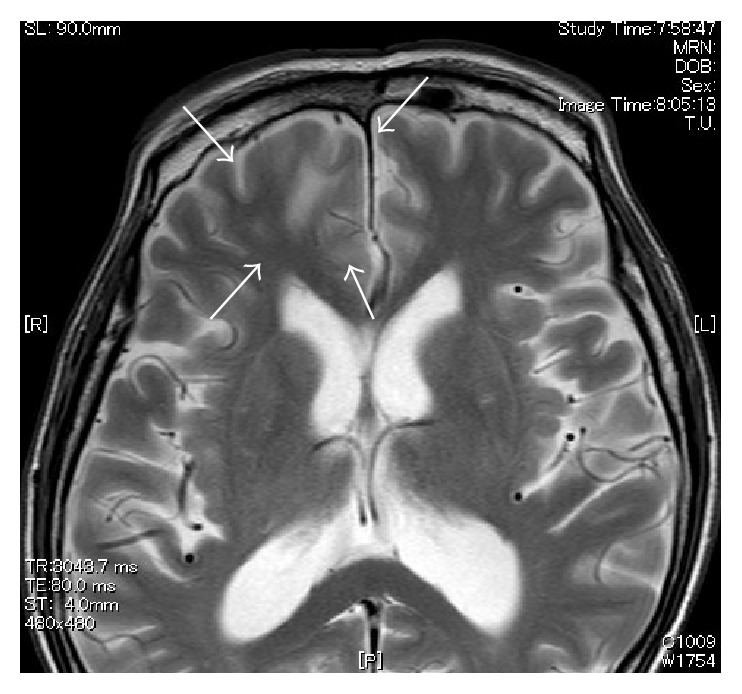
MR image (T2WI) obtained one month after beginning treatment.
